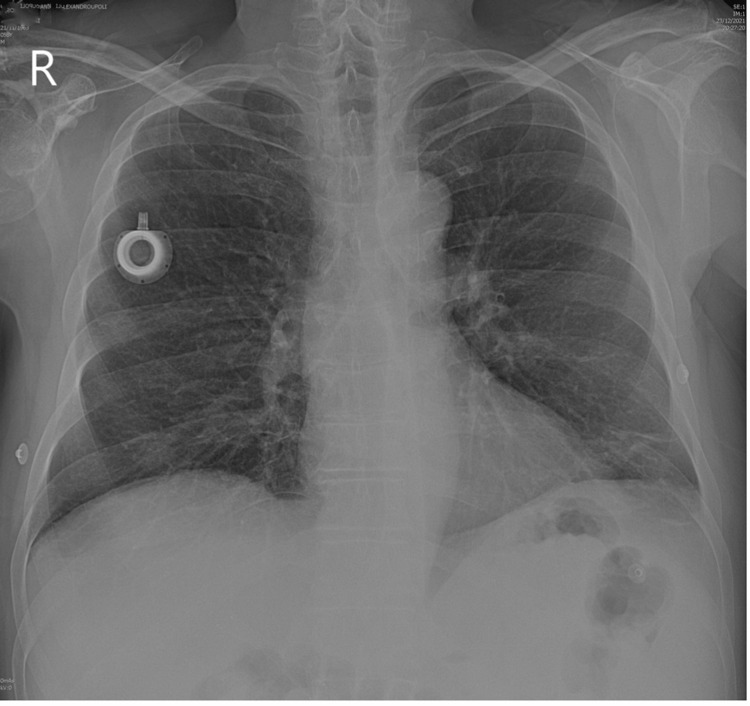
Postoperative chest radiography showing the complete retrieval of the catheter fragment
